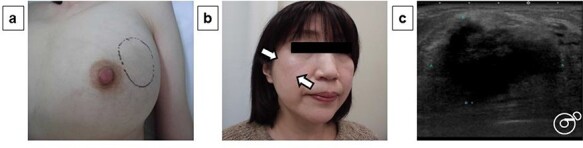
Physical examination and ultrasonography findings: During physical examination, an elastic hard mass measuring ~6 cm in size was palpated in the upper-outer area of the left breast (a). An induration of ~4 cm was also found on the right cheek (b). Mammary ultrasonography revealed an irregular hypoechoic tumor of measuring 45.0 × 43.0 × 32.0 mm in size in the upper-outer quadrant of the left breast (c).
